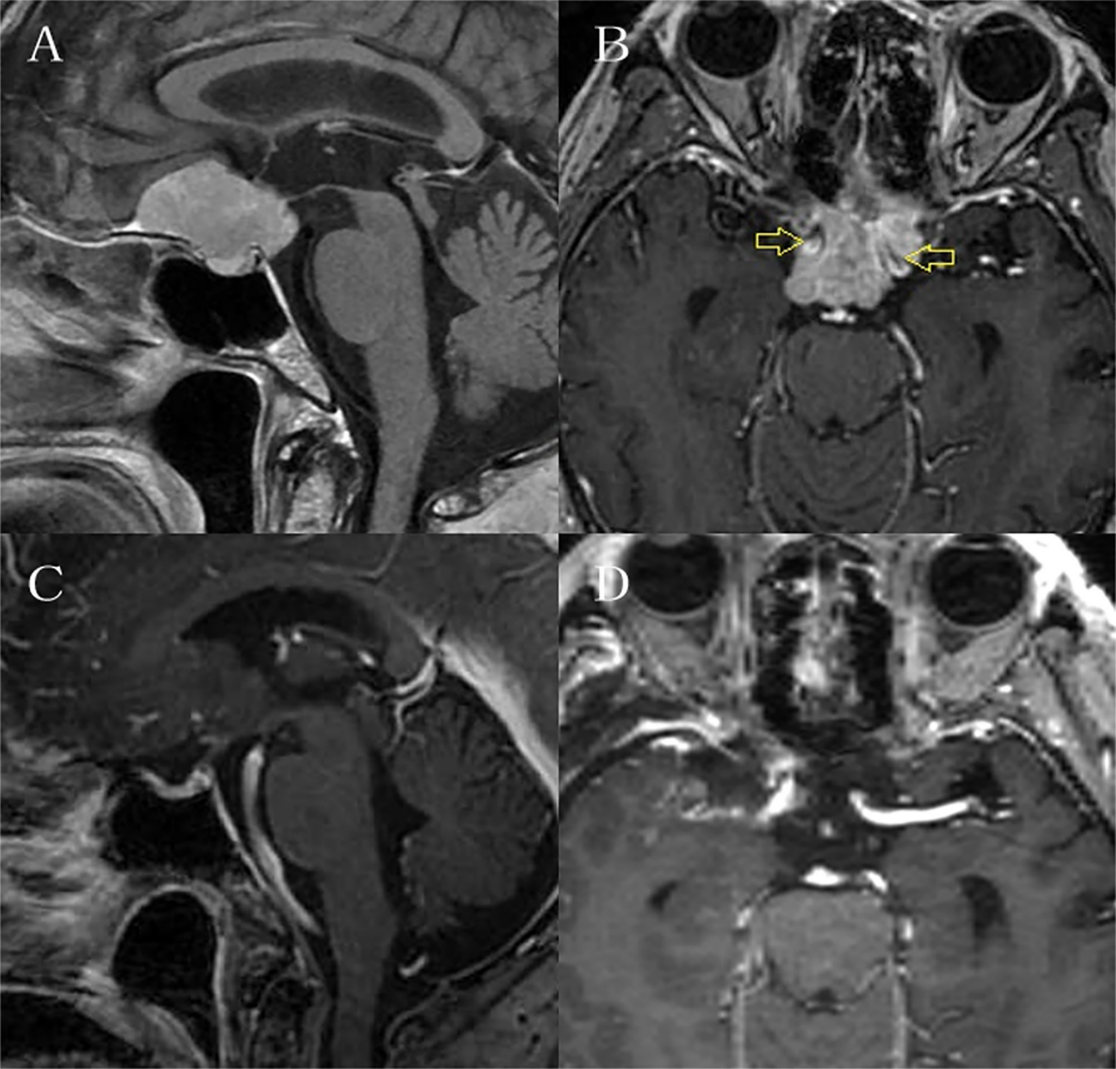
It is the preoperative and postoperative MRI enhancement figure of one case. (A) and (B) showed the tumor was huge and surrounded bilateral internal carotid arteries. (C) and (D) showed total resection of the tumor.
